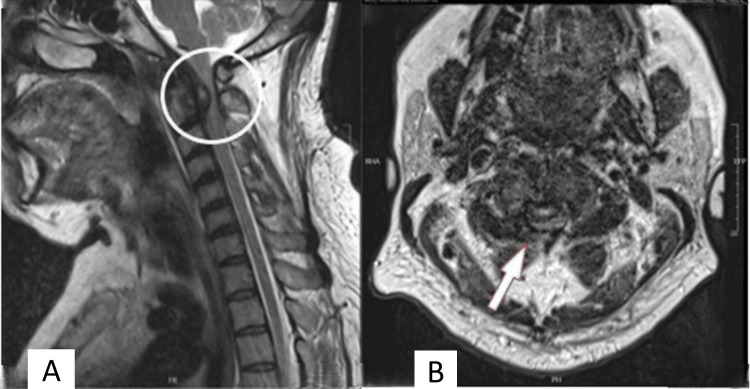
MRI sagittal (A) and axial (B) views of the cervical spine revealed significant cord compression at the level of C2 due to an oval-shaped mass behind the odontoid process (indicated by circle and arrow, respectively).
